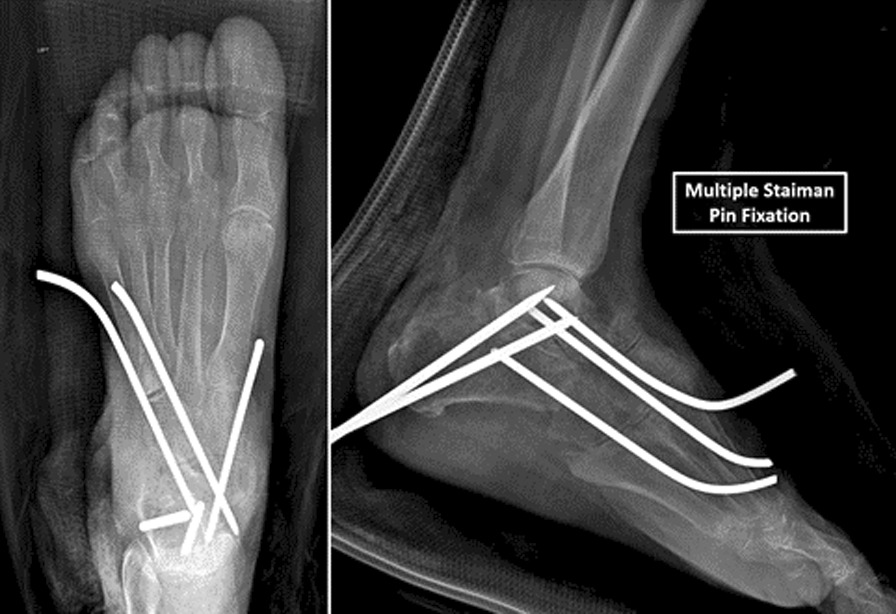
Postoperative x-ray image. Multiple Steinmann pin fixation is seen. In this case, subtalar joint fusion was also performed
